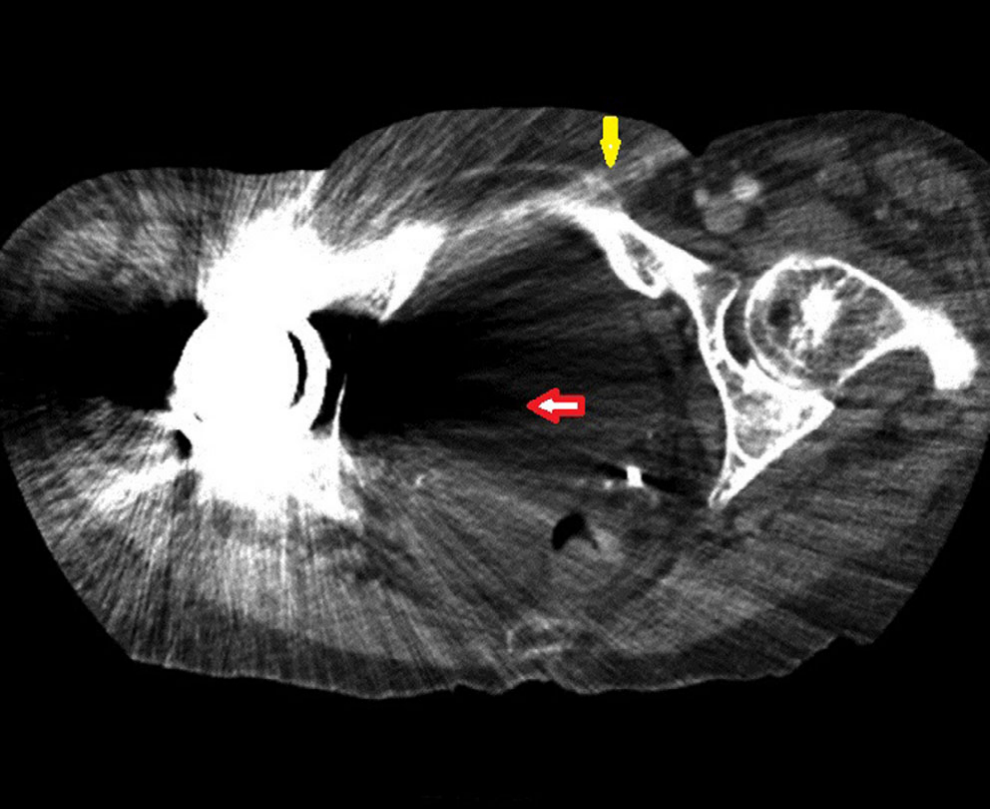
CT scan with artifacts induced by a unilateral hip implant with the appearance of bright streaks (indicated by yellow arrow) and dark streaks (indicated by red arrow).
